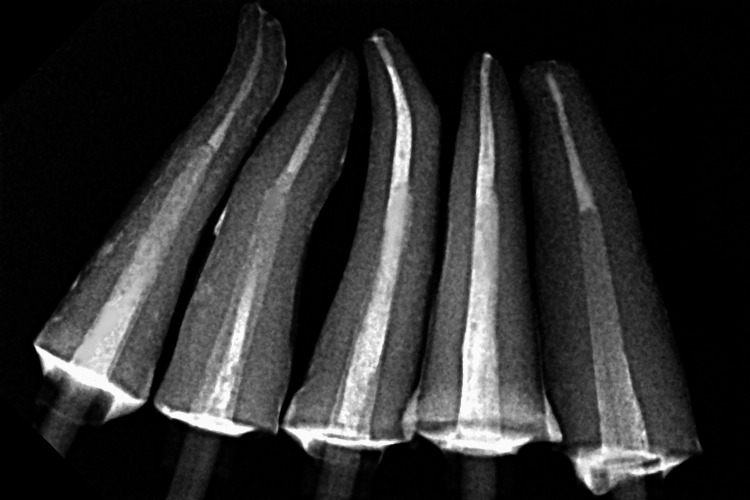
Periapical radiograph after cementation of glass-fiber posts
